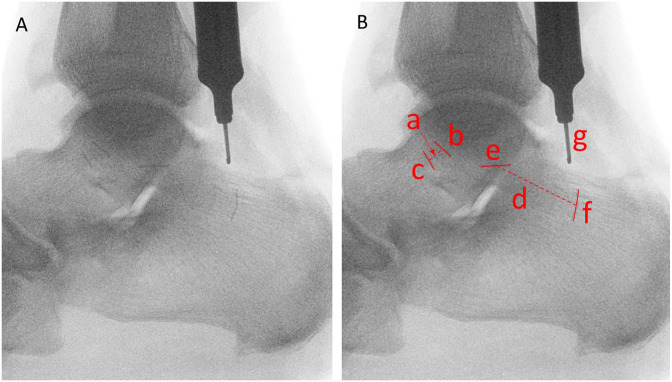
Dimensional measurements for ATFL and CFL. (A) Original radiograph highlighting RAY-TEC wire at the ligamentous footprints on the fibula, talus, and calcaneus. (B) Demonstration of image analysis performed to define ligament dimensions: (a) ATFL length, (b) ATFL fibular footprint, (c) ATFL talar footprint, (d) CFL length, (e) CFL fibular footprint, (f) CFL calcaneal footprint, (g) length-standard depth gauge at 10 mm.
